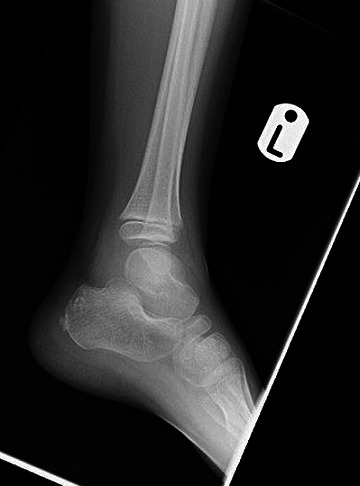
An essentially normal lateral radiograph of the foot demonstrating normal morphology of the talus with no evidence of osteomyelitis, periosteal reaction or evidence of a lytic lesion.
